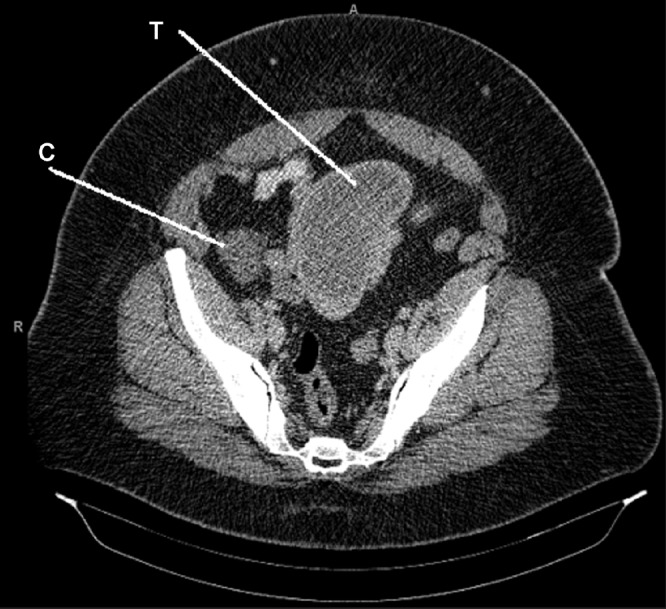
Axial computed tomography of the abdomen demonstrating a tumour (T) in the terminal ileum, which appears thick walled, located close to the caecal pole (C), mimicking an appendiceal abscess
